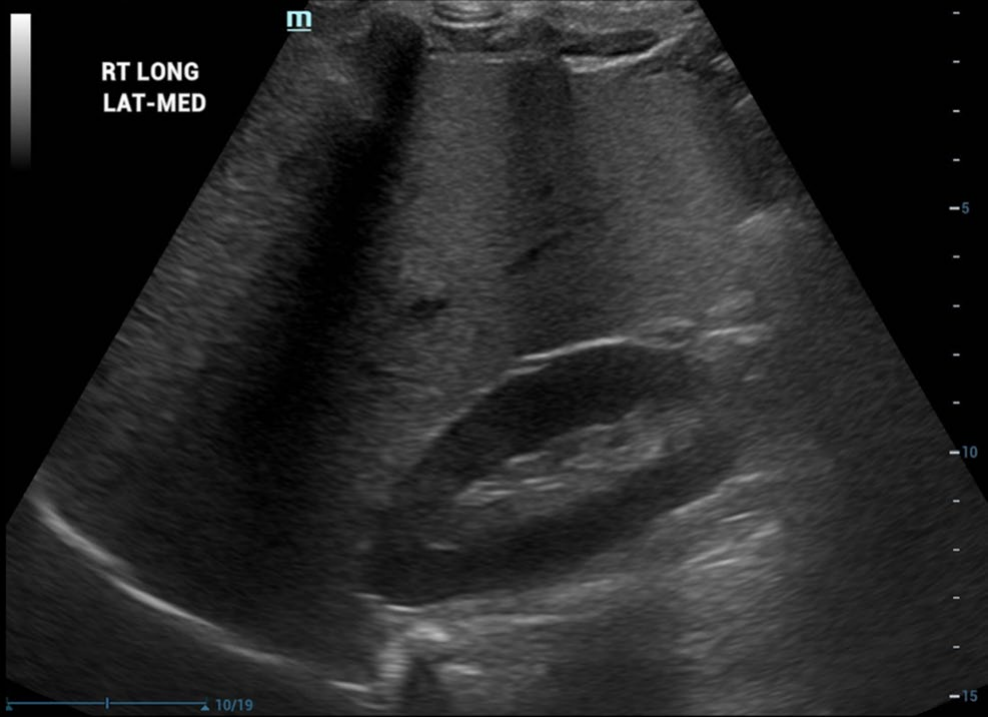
Longitudinal gray‐scale ultrasound image of a 10‐year‐old boy shows echogenic, homogeneous appearance of the liver, consistent with hepatic steatosis. The liver parenchyma is brighter than the cortex of the right kidney.
